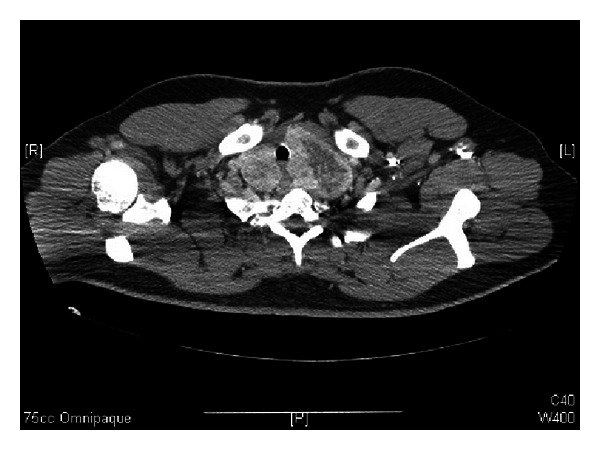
Axial CT scan with contrast at the level of T2, showing an enlarged thyroid gland, larger on the left, with tracheal deviation and inhomogeneous enhancement.
